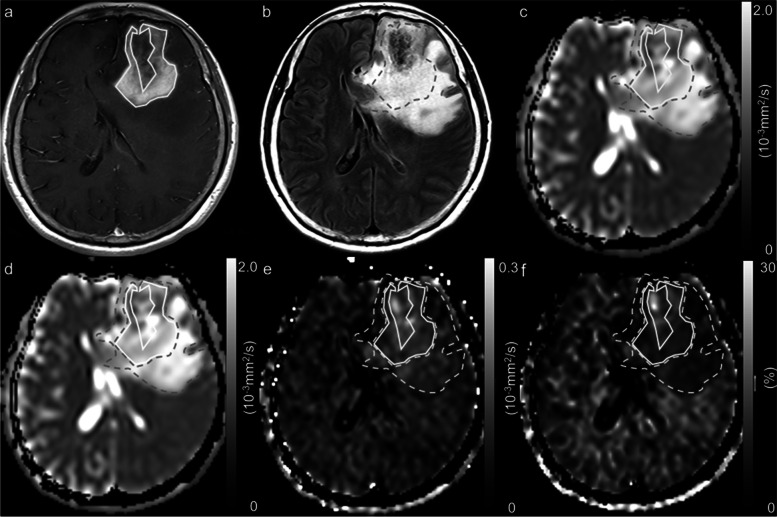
A 65-year-old woman with glioblastoma, isocitrate dehydrogenase-wildtype, grade 4. A contrast-enhanced T1-weighted image with a ROI (solid line) (a), a fluid attenuated inversion recovery image with a ROI (dotted line) (b), an ADC map derived from pulsed gradient spin-echo (PGSE) DWI at an effective diffusion time (Δ_eff_) of 44.5 ms (c), an ADC map derived from oscillating gradient spin-echo (OGSE) DWI at an Δ_eff_ of 7.1 ms (d), and maps of the ADC change between PGSE DWI and OGSE DWI (cADC) (e) and the relative ADC change between PGSE DWI and OGSE DWI (rcADC) (f). The ADC values in the tumor appear higher at short Δ_eff_ values than at long Δ_eff_ setting. Small changes in the cADC and rcADC are noted between the OGSE and PGSE sequences in the tumor
